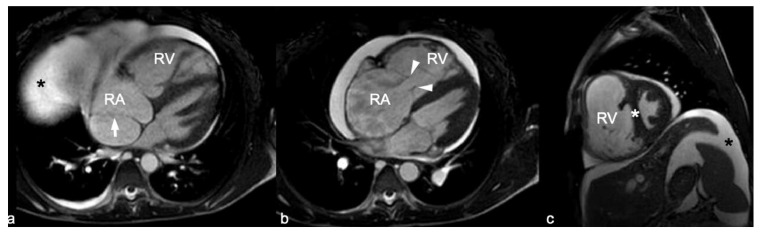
SSFP Cardiac MR images in the 4 chamber (a,b) and short axis (c) planes. RV and RA are severely dilated and diastolic septal shifting (white asterisk on c) as a sign of volume overload of the RV are seen. The eustachian valve is seen in the RA (White arrow on a) with no other membranous structures seen on more cephalic images. Severe tricuspid regurgitation with significant coaptation defect (between white arrowheads in (b)) was found, together with abdominal free fluid (Black asterisk on (a) and (c)), pericardial and pleural effusions. SSFP: Steady State Free Precession.
